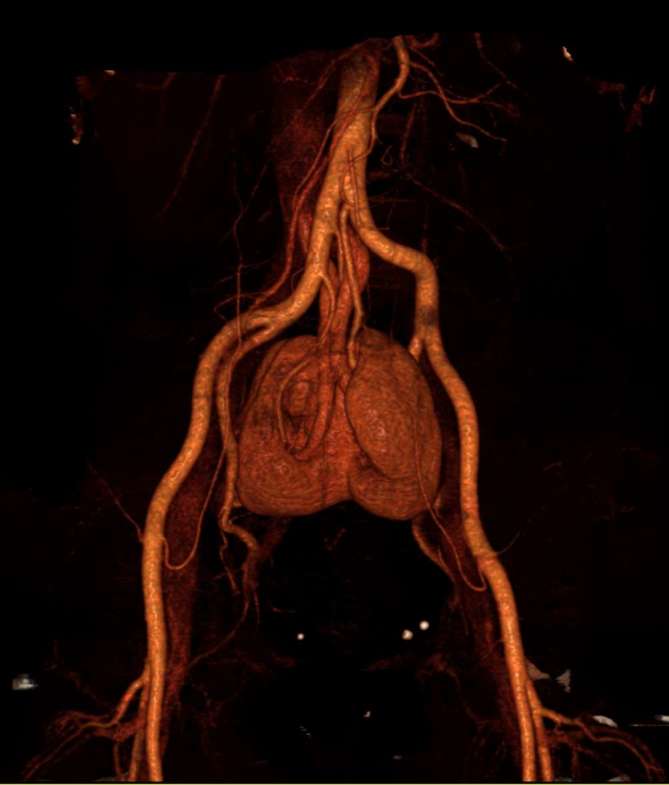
CT 3D volume rendering of vascular supply to pancake kidney: dual arteries
